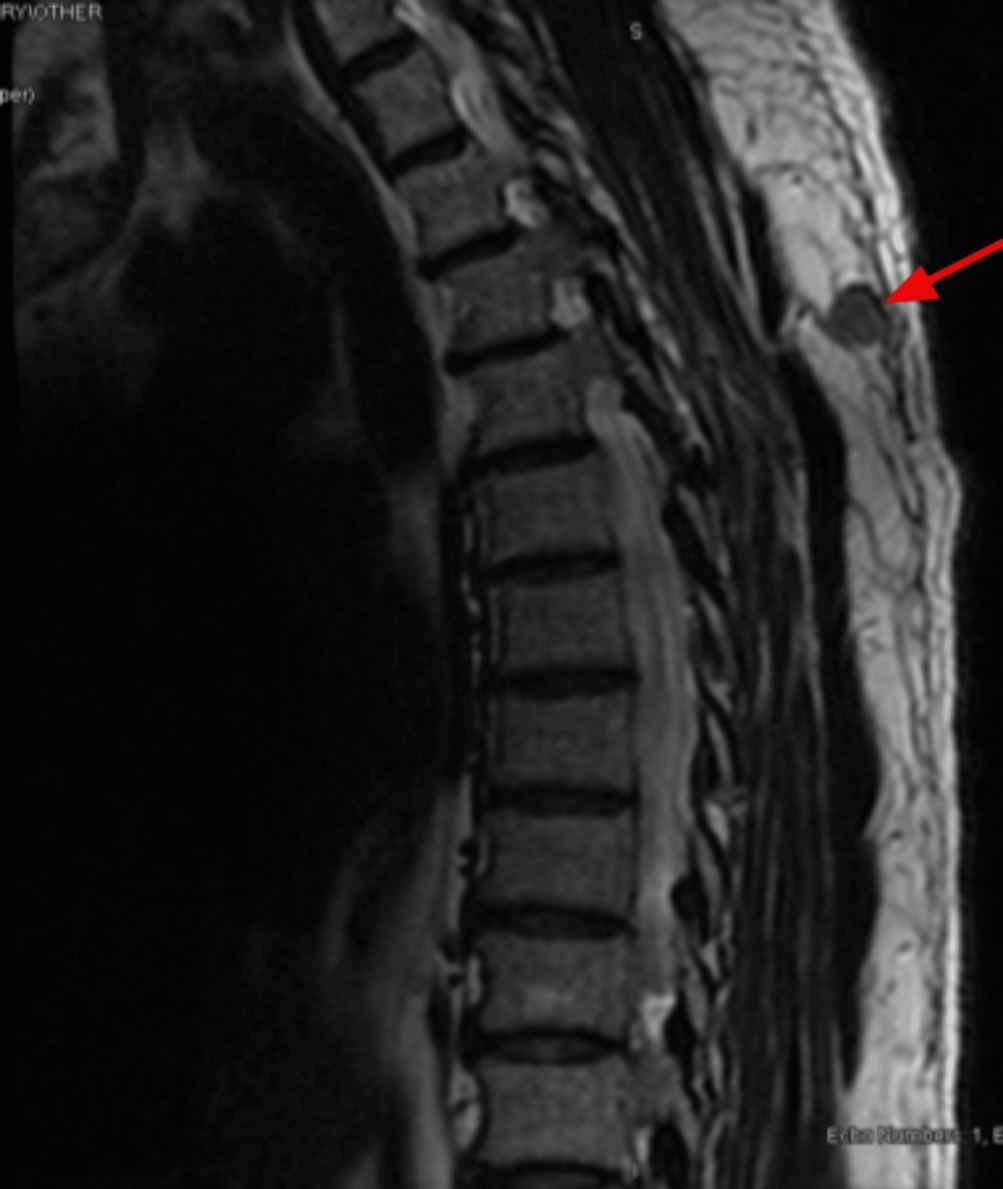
Spinal MRI (April 12, 2023) showing an enhancing hemorrhagic lesion in the soft tissue
